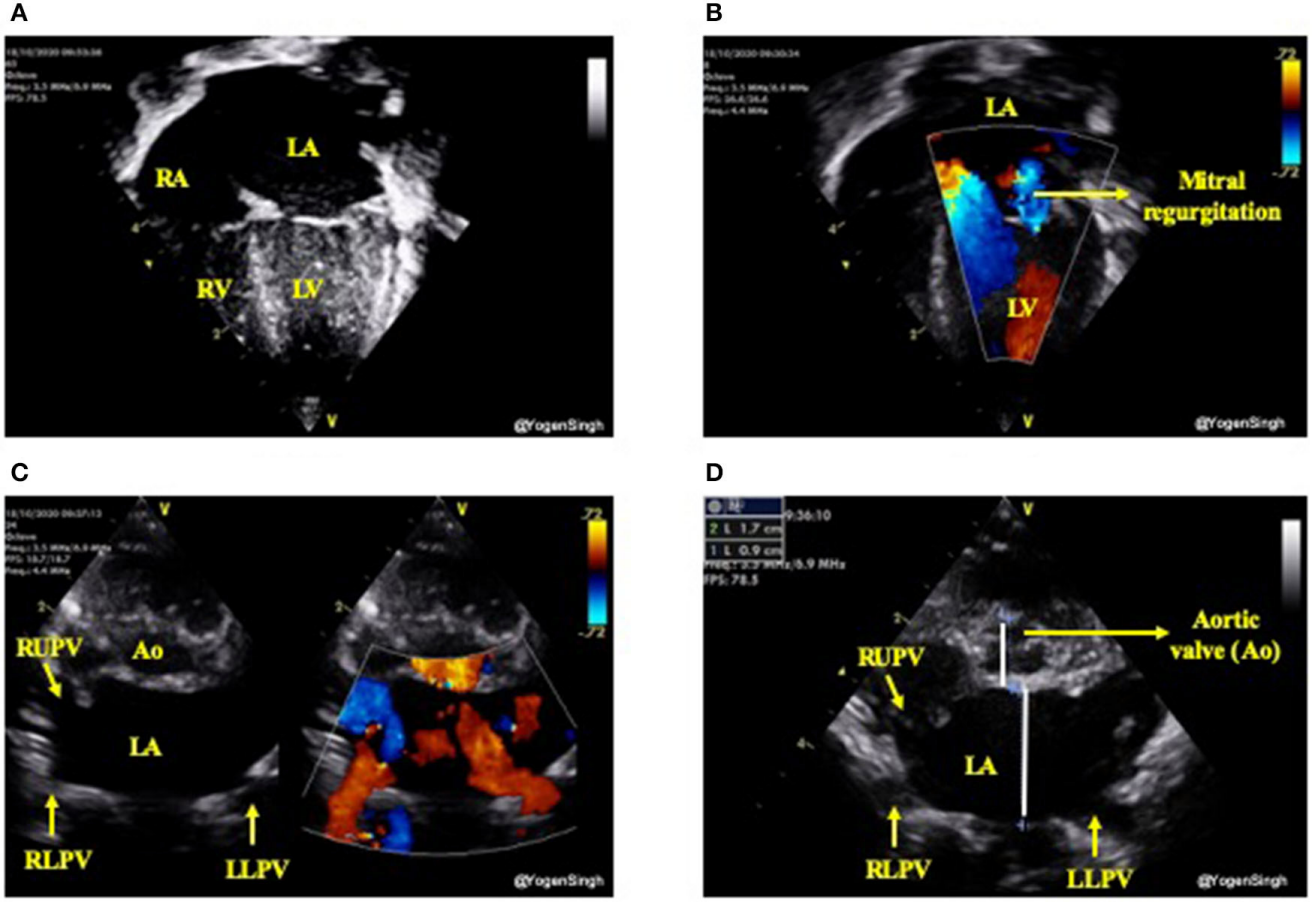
Assessment of left heart volume overloading on visual inspection “eyeballing.” (A) Apical 4 chamber view in 2D showing dilated left side of the heart (dilated left atrium and left ventricle); (B) Mitral regurgitation on color flow mapping as blue jet going back to left atrium (see explanation in text); (C) “Crab view” showing dilated pulmonary veins reflecting increased pulmonary venous return and (D) Dilated left atrium in parasternal short axis view—on visual inspection LA looks almost the double the size of aortic valve (Ao). LA, left atrium; LV, left ventricle; RA, right atrium; RV, right ventricle; Ao, aortic valve; RUPV, right upper pulmonary vein; RLPV, right lower pulmonary vein; LLPV, left lower upper pulmonary vein.
